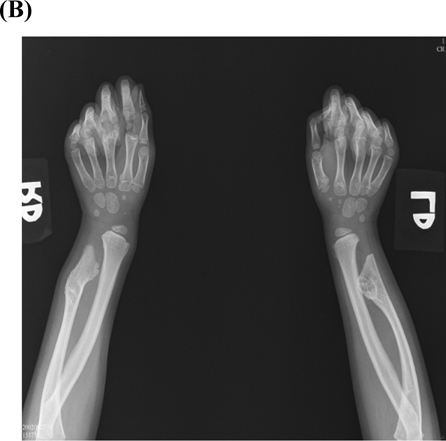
Photograph and radiograph of Patient #1. (A) Both forearms and (B) X-ray image. Note bowing of radius and ulna with bony exostosis over distal ulna.
